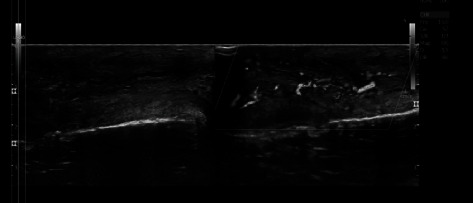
Ultrasound of the penis showing increased echogenicity on the left side of the penile shaft compared to the right side of the shaft.
